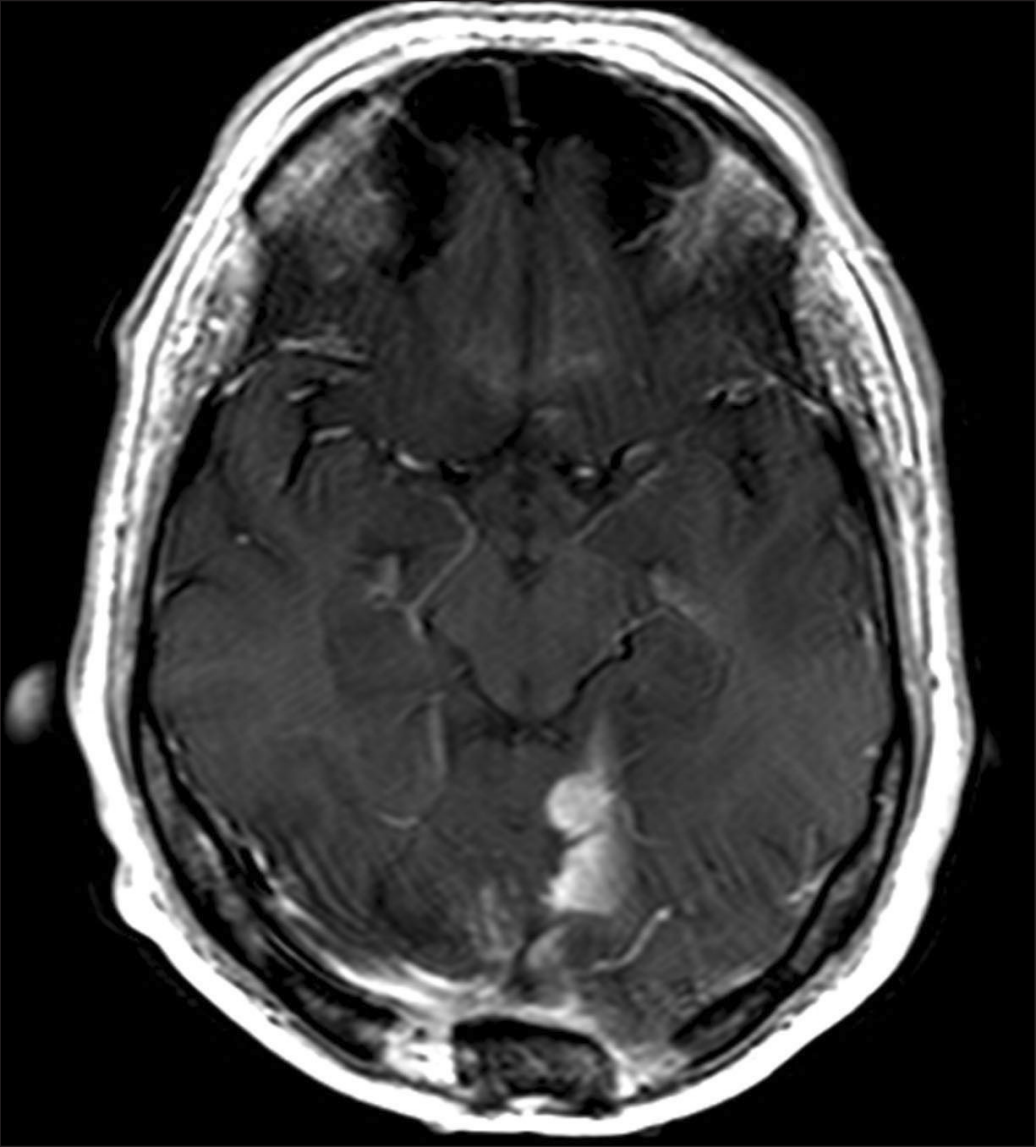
Axial T1-weighted MRI with gadolinium enhancement showing recurrence of lesion
